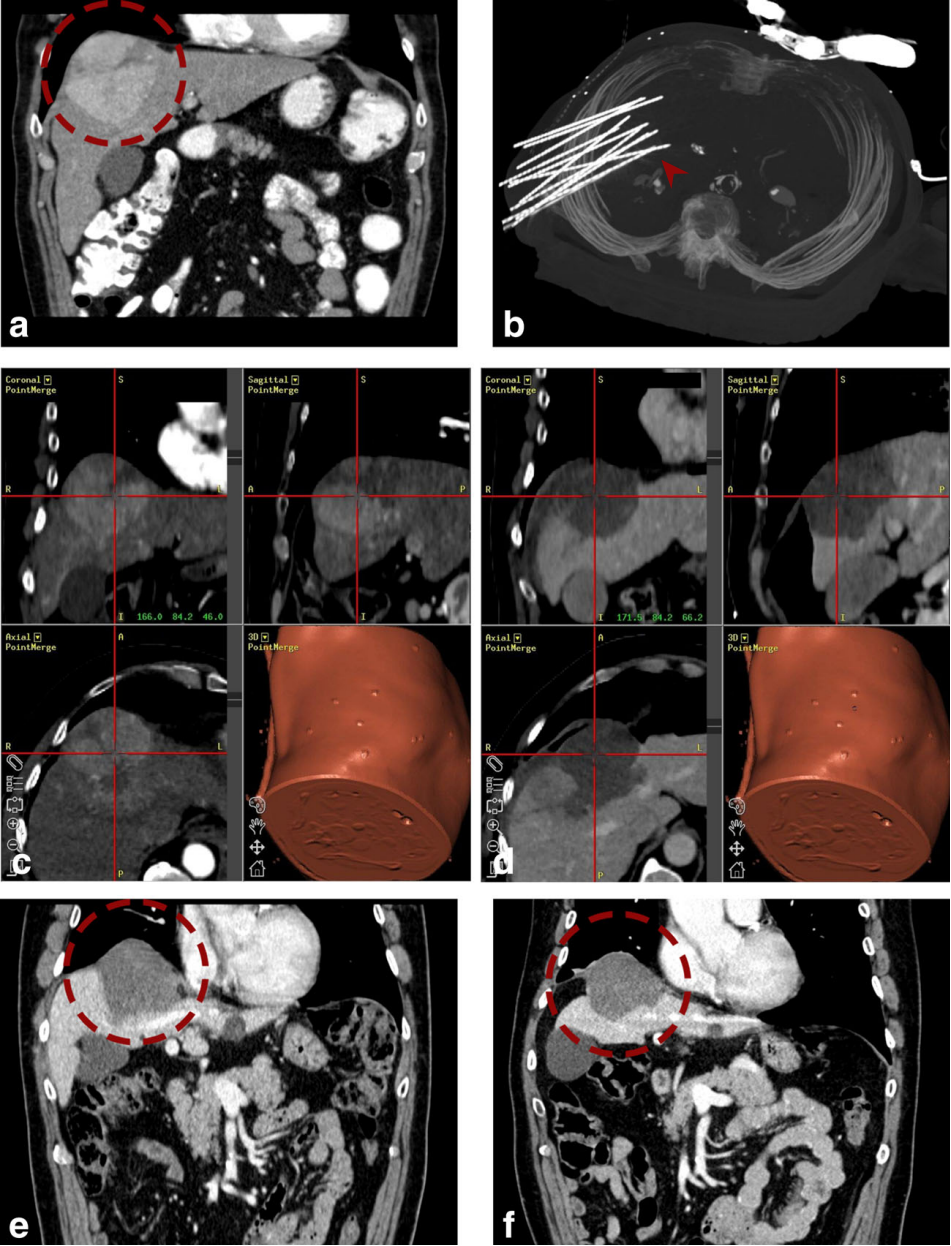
Case of a 67-year-old male with a large HCC in the hepatic dome. a Arterial phase planning CT with a 9-cm HCC in segment IV/VIII (*red dashed circle*). b MIP of the control CT showing in total 12 inserted coaxial needles (*red arrowhead*). c, d Fused images from the navigation system with 3D views from arterial phase planning CT (a) and final control CT (d) with complete necrosis including a sufficient ablation margin. e, f Follow-up CT scans after 3 (e) and 24 months (f) with no evidence of local tumor recurrence (*red dashed circle* is marking the coagulation zone)
